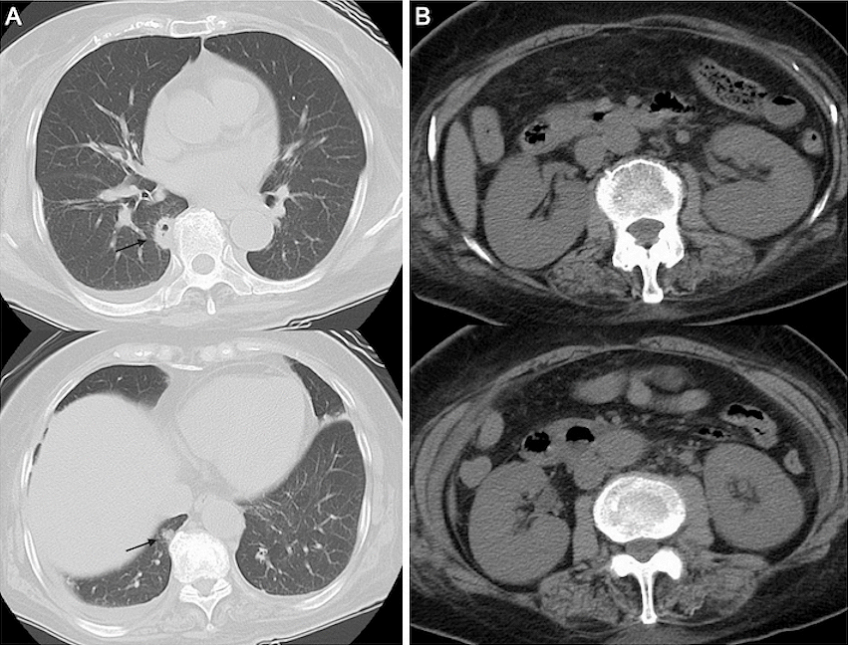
(A) Computed tomography (CT) of the chest revealed multiple small nodular infiltrates with cavities (arrow). (B) Abdominal CT revealed the enlargement of both the kidneys.
